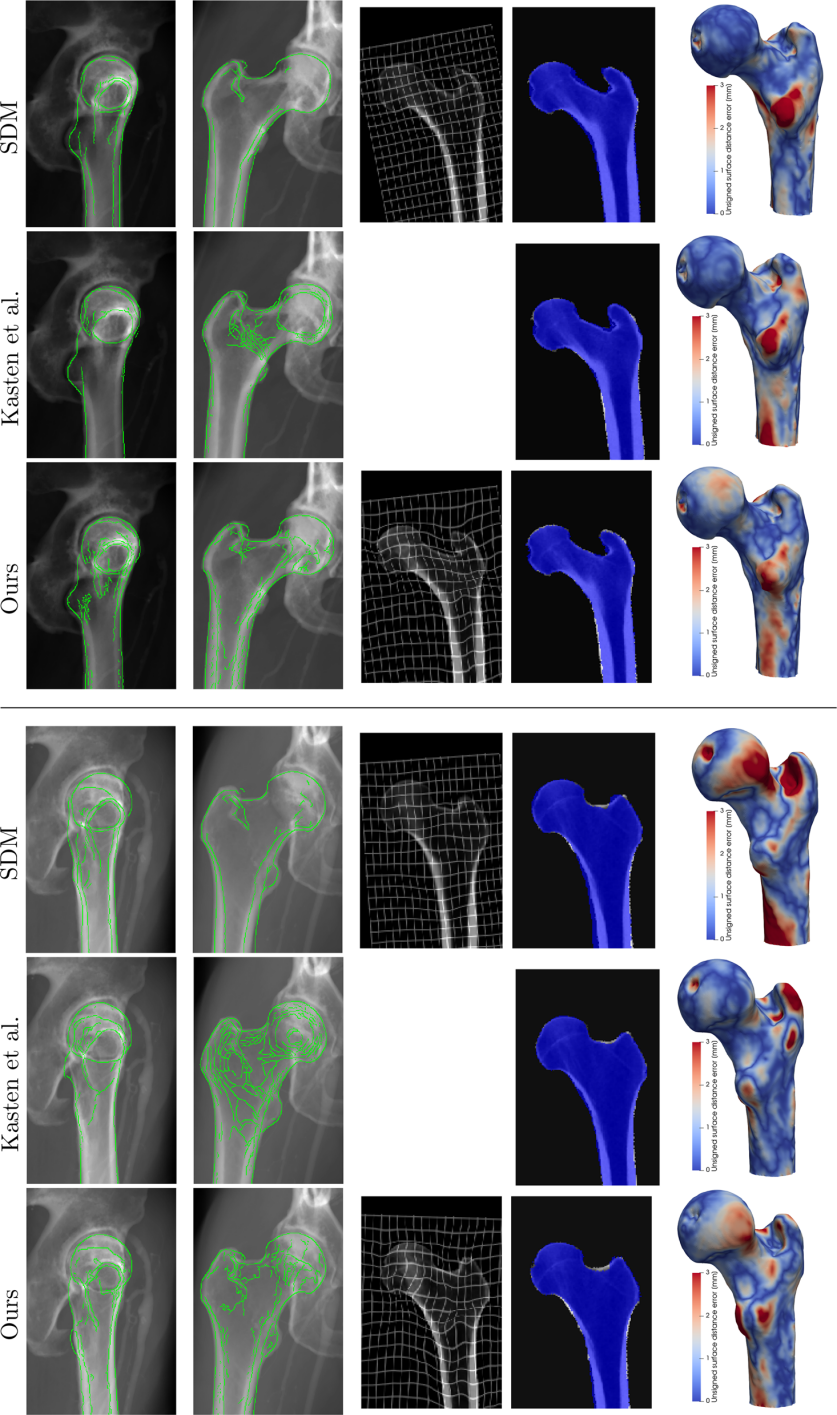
Examples of 2D/3D registration based on AP and lateral radiographs by different DL models. The first two columns show the lateral and AP input radiographs overlapped with the contours of the DRRs from the predicted 3D image volume. The third column shows a coronal slice of the warped atlas volume with the deformation grid. The fourth column shows a coronal slice of the predicted segmentation map, overlaid on top of the ground-truth image. The last column shows the geometric reconstruction error between the reconstructed and ground-truth surface model
